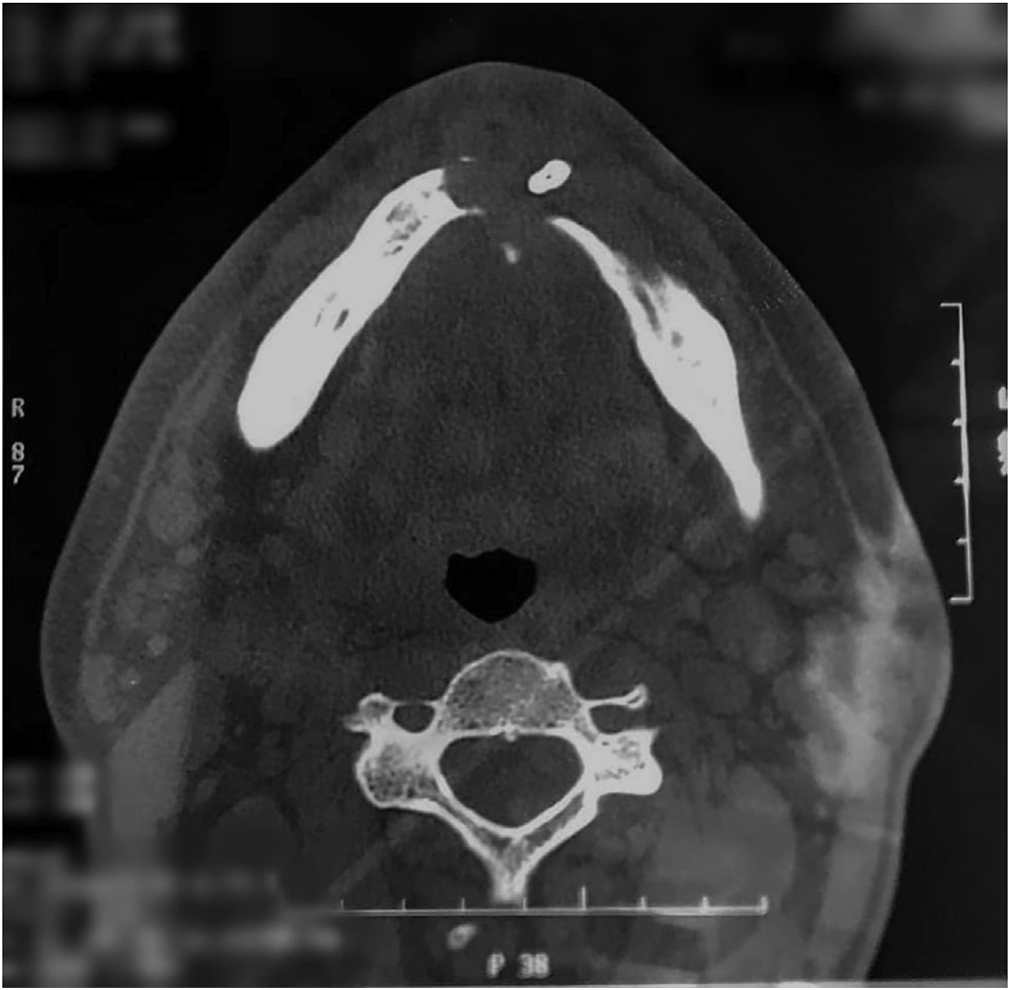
CT scan with an axial section showing the mandibular cortical interruption secondary to an osteolytic lesion.
